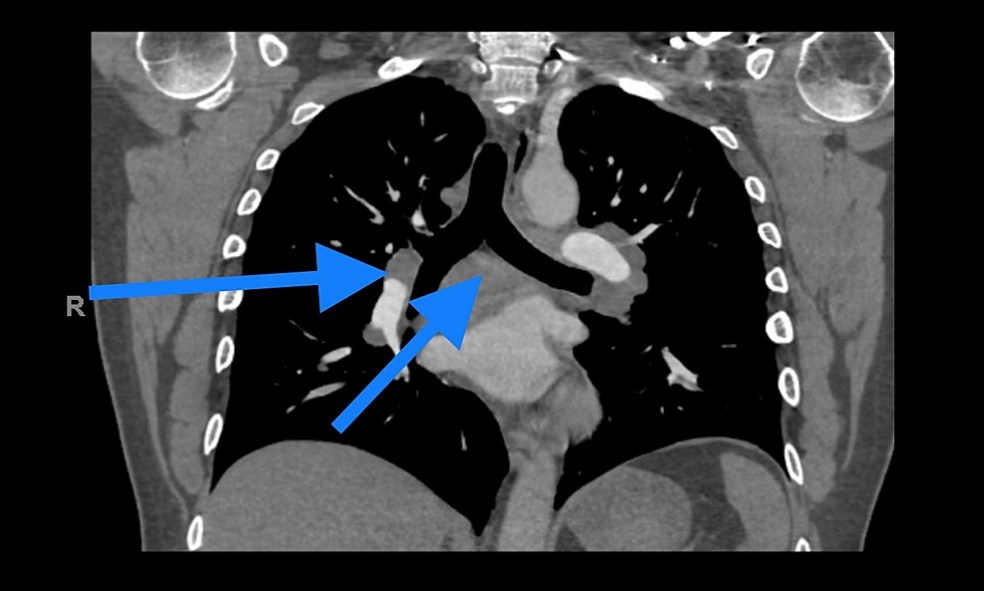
CT with IV contrast showing bilateral mediastinal and hilar lymphadenopathy. CT: computed tomography; IV: intravenous
